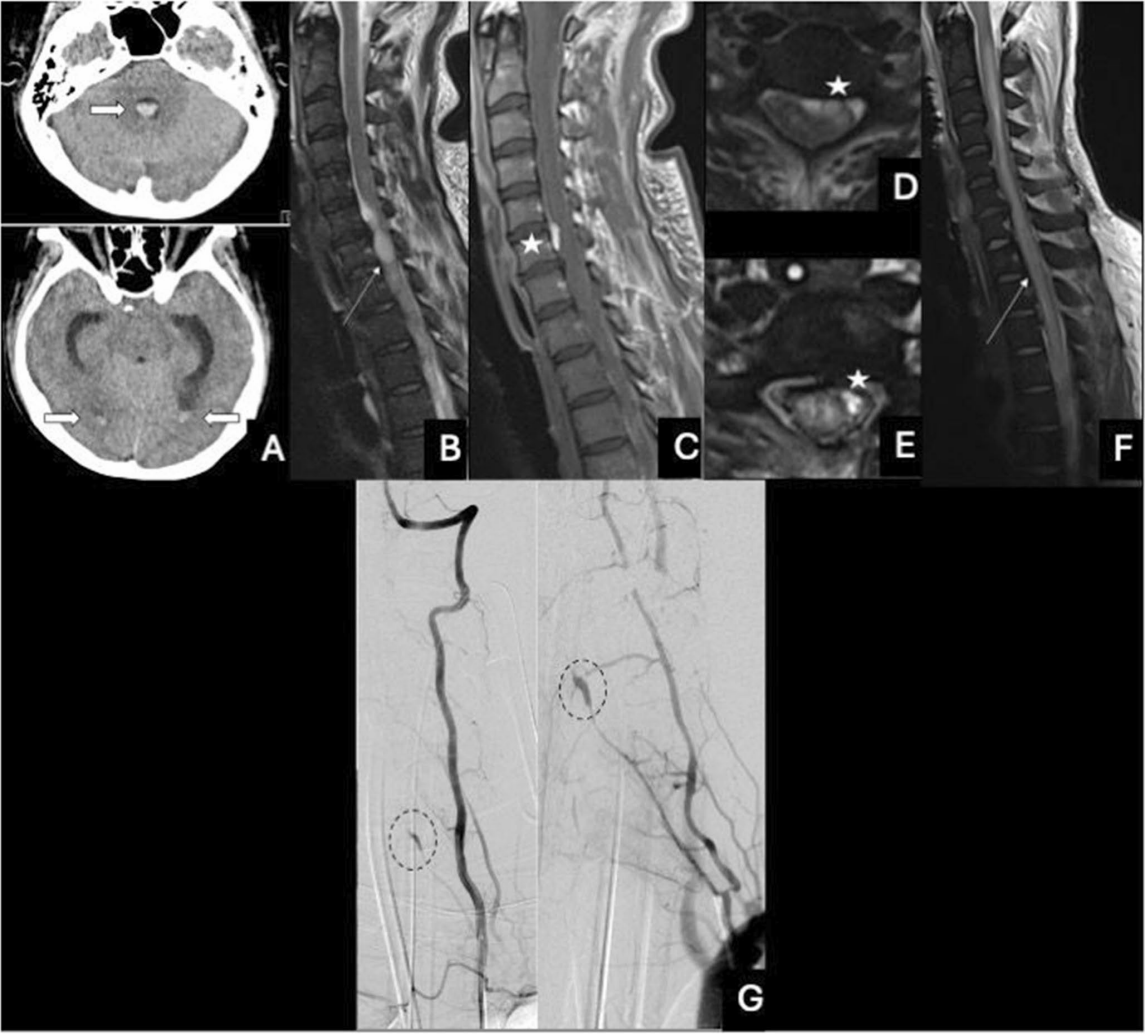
Representative case (Patient 1). A) Head CT showed minimal basal SAH. B-F) Spinal MRI showed extensive cervical and intracranial SAH as seen on sagittal T2 (B) and axial T2 (D) weighted images, as well as an intradural cystic mass ventral to the cervical myelon as seen on sagittal (C) and axial (E) contrast-T1 weighted images. Extensive spinal cord infarction is seen on sagittal T2 (F). A fusiform aneurysm of a cervical RMA at C7 level was found on DSA, with supply from the left inferior thyroid artery and the cervical ascendent artery (G)
